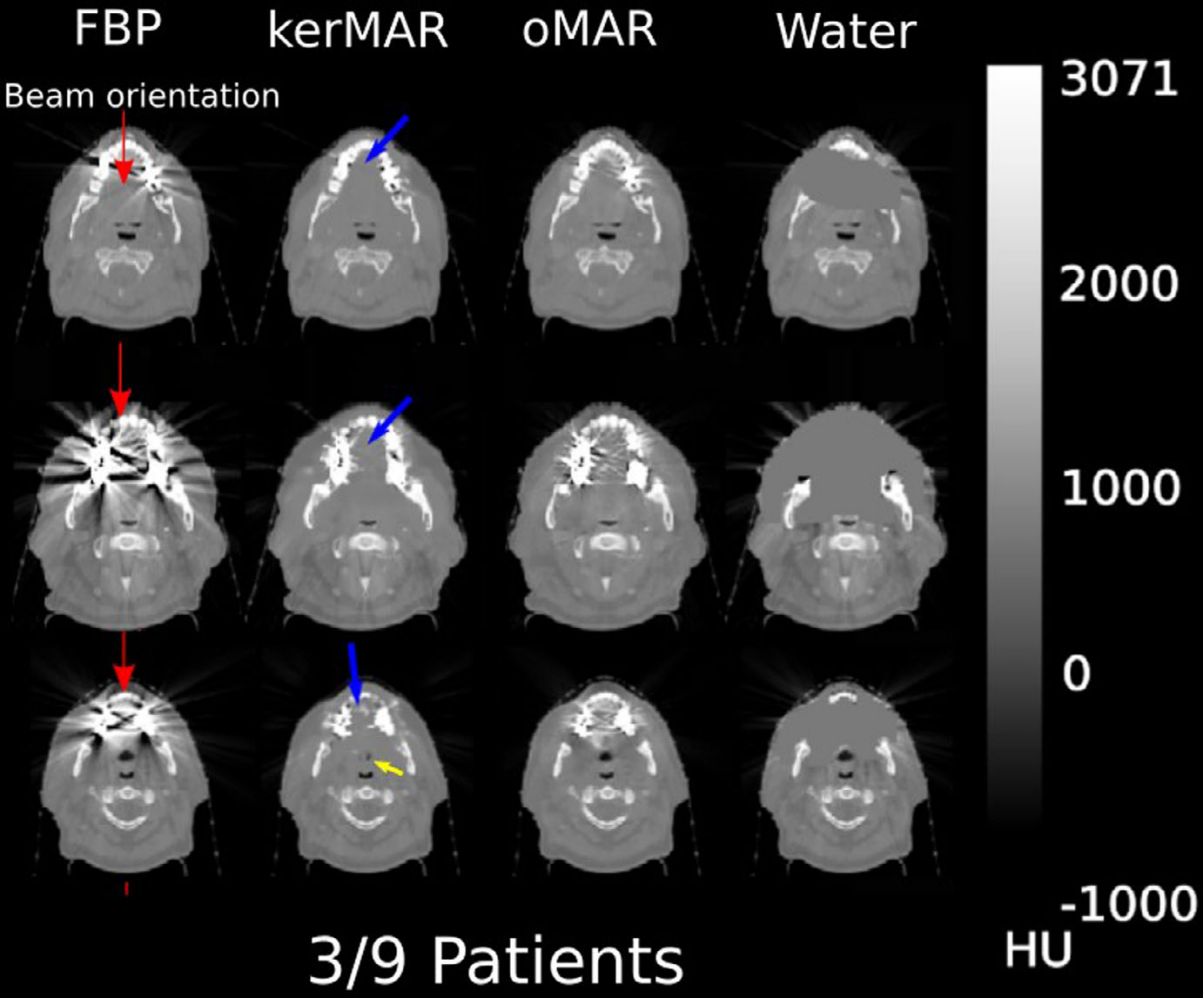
Axial filtered back projection (FBP) and metal artifact reduced slices in 3 of 9 patients. Red arrows as in Fig. 1. Blue arrows indicate potential benefits of kernel regression metal artifact reduction (kerMAR) over metal artifact reduction for orthopedic implants (oMAR). The yellow arrow indicates kerMAR‐introduced artifacts near the trachea caused by different magnetic resonance and computed tomography acquisition positions. [Color figure can be viewed at http://www.wileyonlinelibrary.com/]
